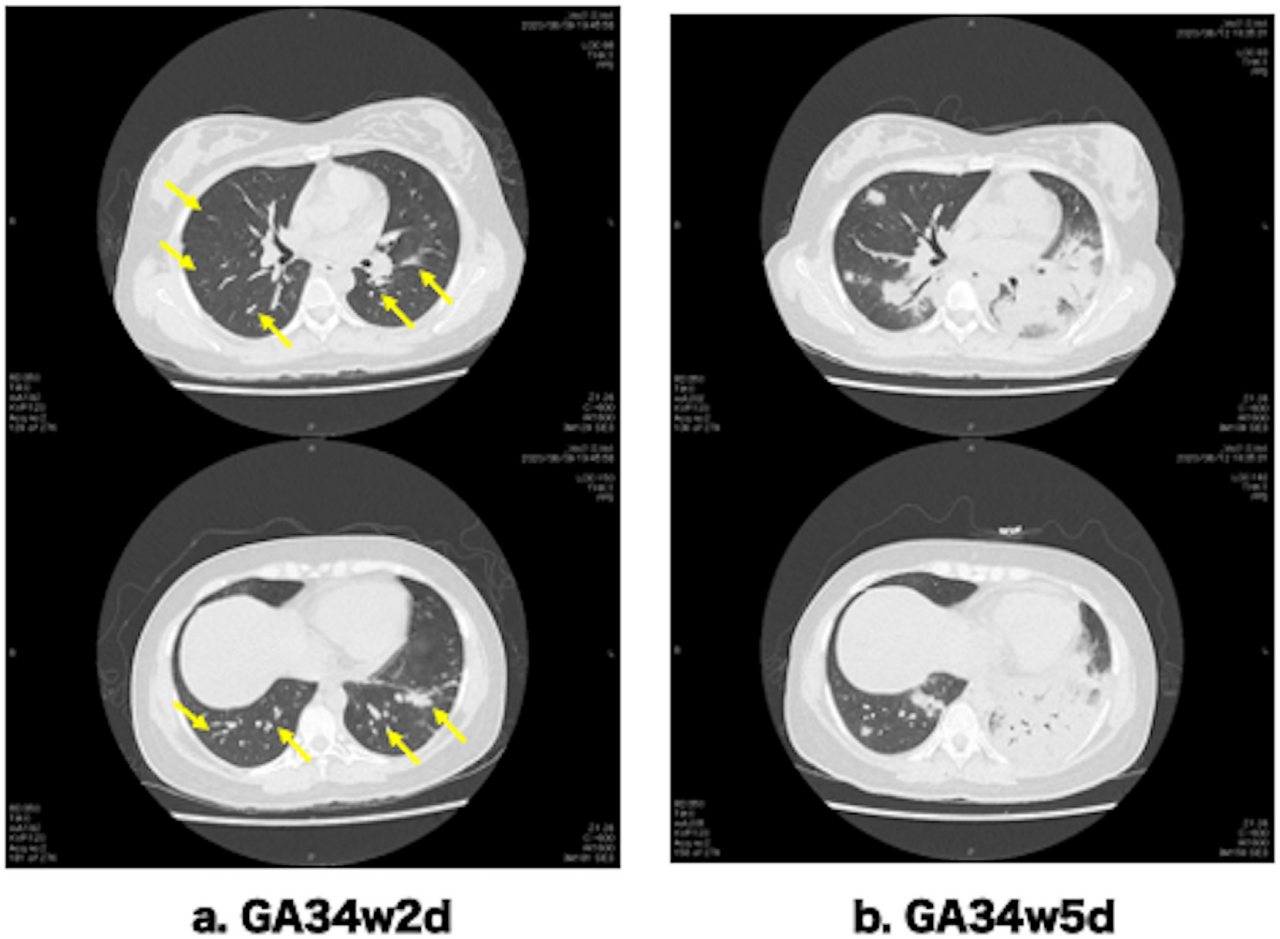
(a) On admission of the patient at 34 weeks and 2 days of gestation, a chest computed tomography scan revealed bilateral multifocal ground-glass opacities with partial consolidation (arrows). (b) Chest computed tomography at 34 weeks and 5 days of gestation showed rapid deterioration.
